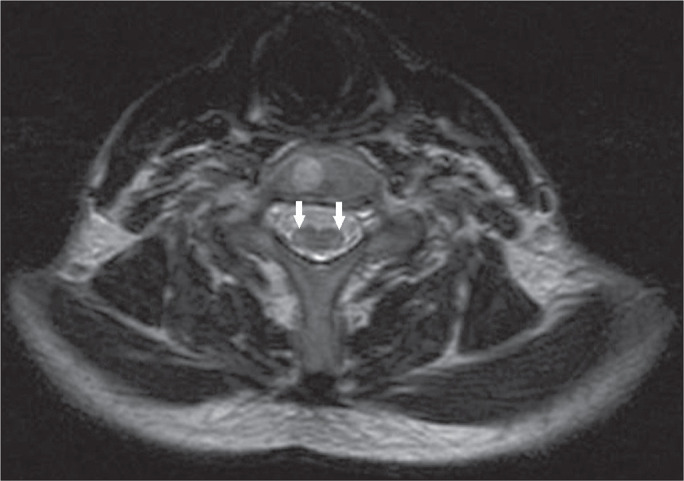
AIDS-associated myelopathy. Axial T2-weighted MRI sequence showing a
